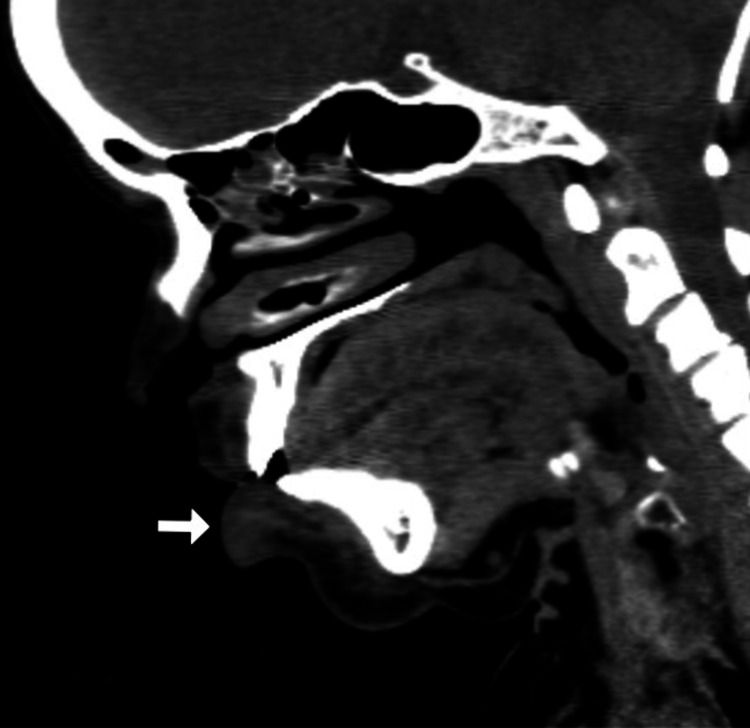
CT of the head and neck (sagittal view) showing diffuse soft tissue swelling in the lower lip (white arrow) CT: computed tomography
